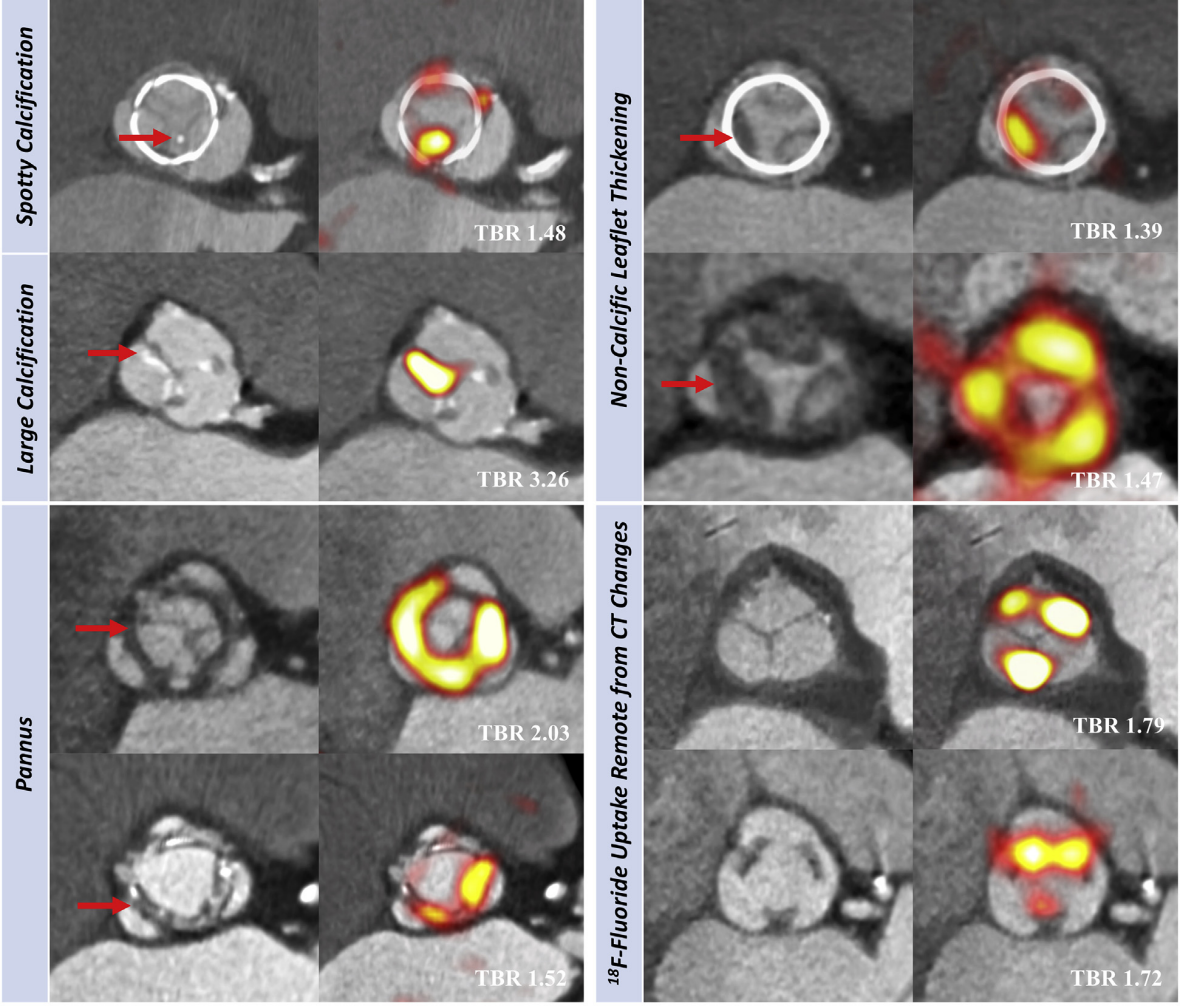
In Vivo ^18^F-fluoride PET and CT Imaging of Patients With Bioprosthetic Aortic Valves Baseline CT (left) and ^18^F-fluoride PET (right) images from patients with bioprosthetic aortic valves. En face CT images of aortic bioprosthetic valves showing spotty calcification and large calcification (top left), circumferential pannus (bottom left), and noncalcific leaflet thickening suggestive of thrombus (top right) (all abnormalities identified by red arrows). Hybrid en face PET-CT images in the same patients: increased bioprosthetic ^18^F-fluoride activity (red/yellow areas) is observed in each patient colocalizing with the CT abnormalities. ^18^F-fluoride activity was also commonly observed remote from leaflet changes on CT (bottom right). Target-to-background (TBR) values are annotated on the hybrid PET-CT images in white text. Abbreviations as in Figure 1.
